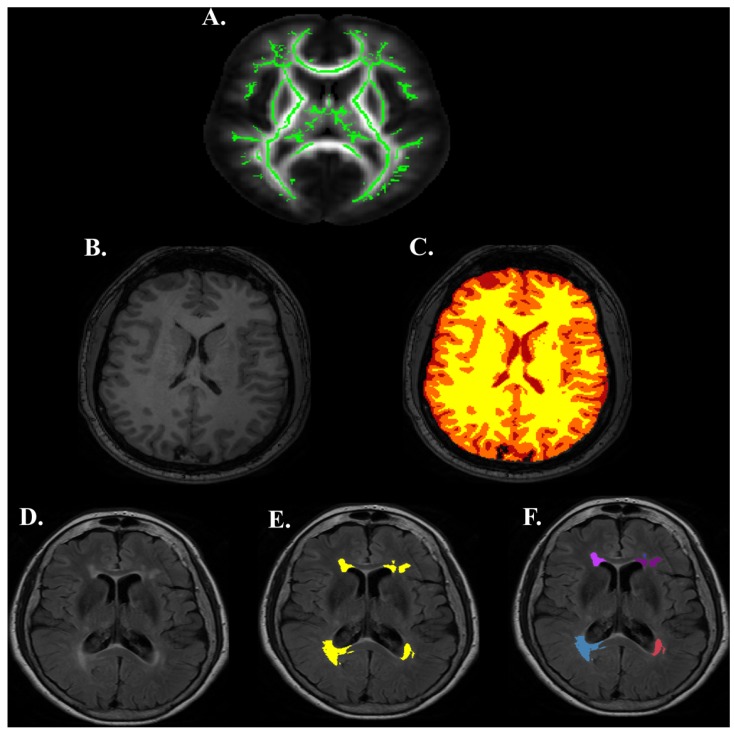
Diffusion and Structural Image Processing Examples. (A) Diffusion tensor imaging white matter skeleton. (B) T_1_ image. (C) T_1_ image segmented into white matter (yellow), grey matter (orange), and cerebral spinal fluid (red) using SIENAX. (D) T_2_-FLAIR image. (E) Lesions demarcated (yellow) on T_2_-FLAIR image used for calculating lesion burden. (F) Spatially distinct lesions demarcated on T_2_-FLAIR image.
